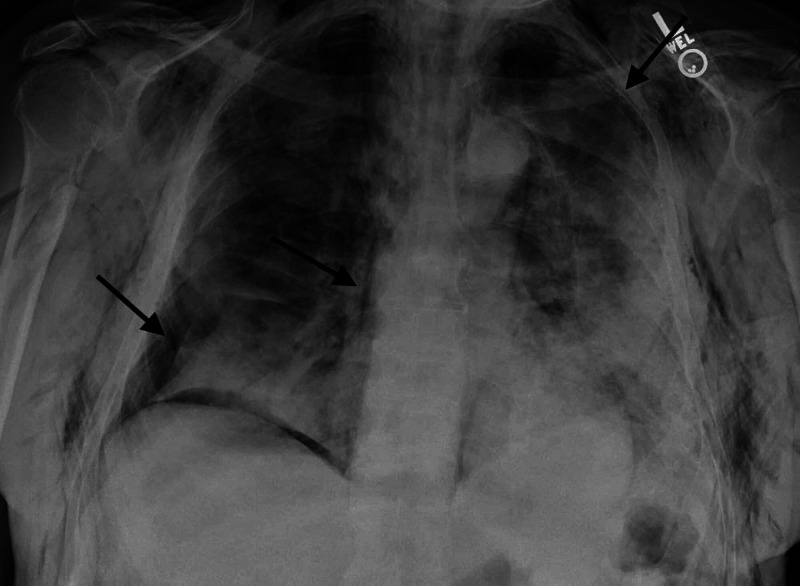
Chest X-ray shows bilateral pneumothoraces as well as pneumomediastinum as shown by arrows
